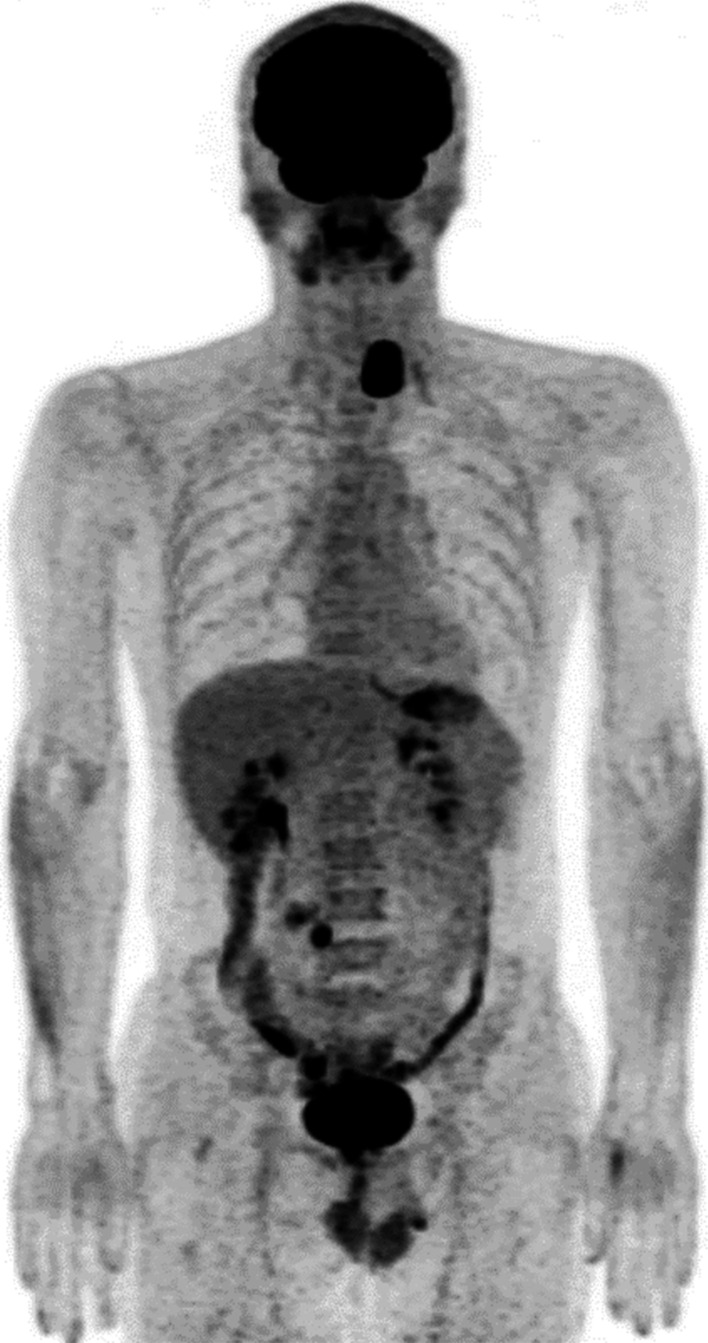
PET–CT. Abnormal accumulation is seen in the left thyroid lobe (SUVmax, 23.90), no other abnormalities
